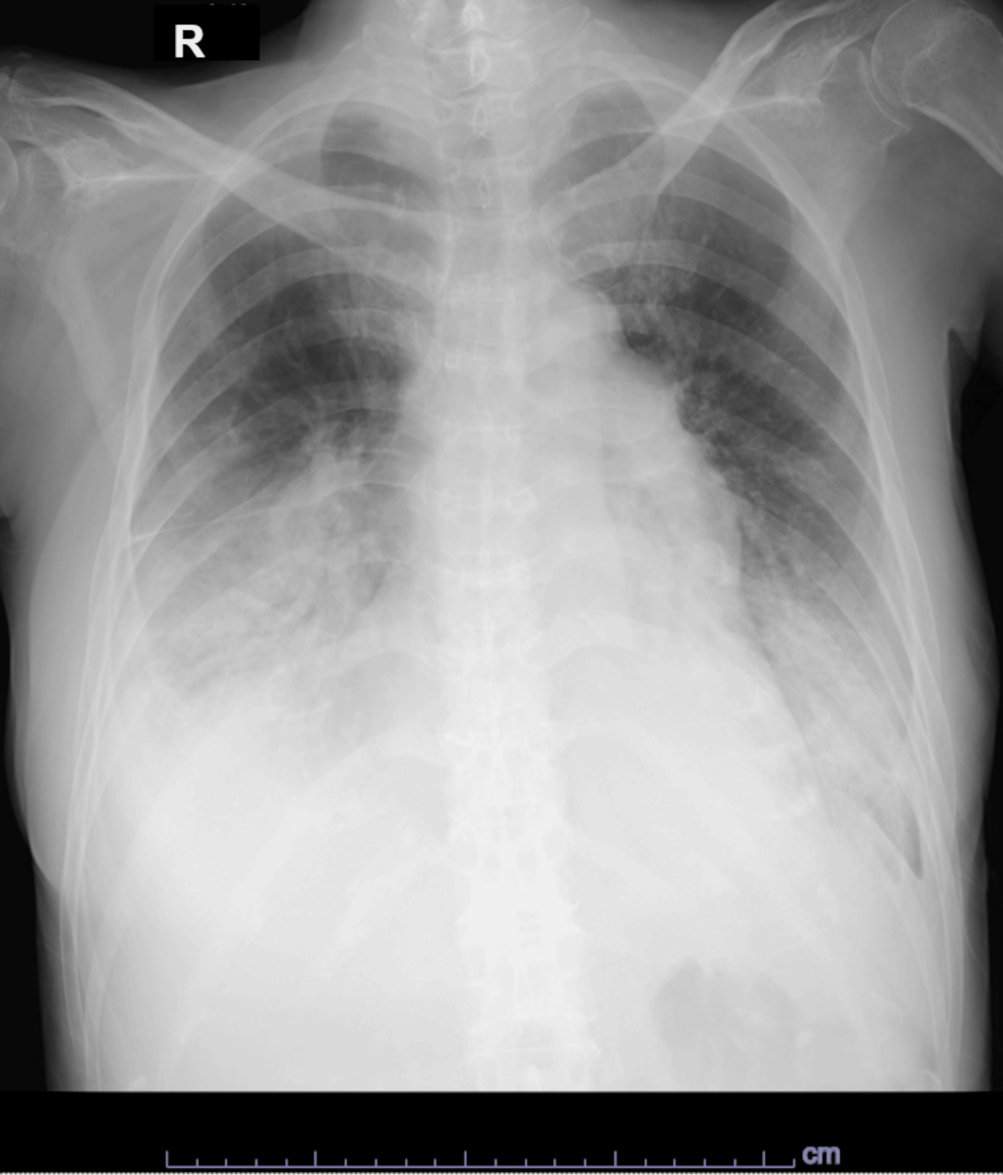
Findings of chest radiography. Bilateral pleural effusions were observed.
